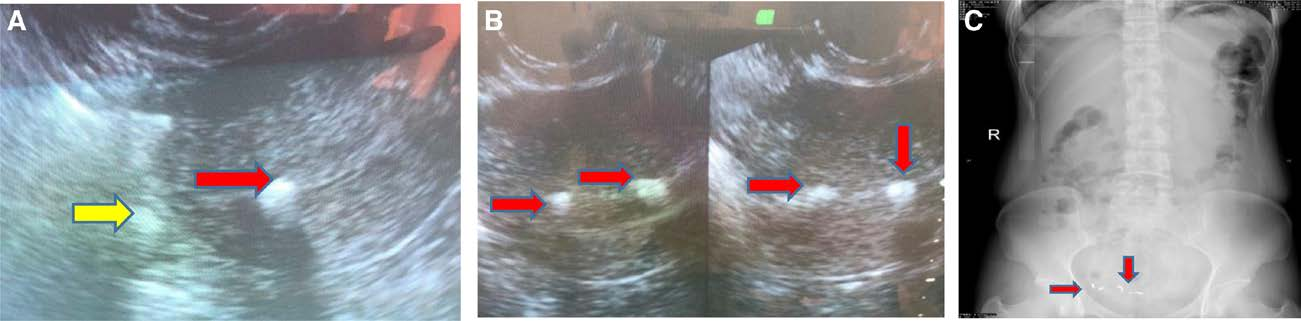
(A) Metal-like hyperechoic spots within (red arrow) and outside of (yellow arrow) the myometrium of the right side of the uterus on ultrasonography. (B) An IUD normally positioned in uterine cavity (red arrow) on ultrasonography. (C) Two irregular high-density shadows in the pelvic cavity (red arrow) on abdominal X-ray.
